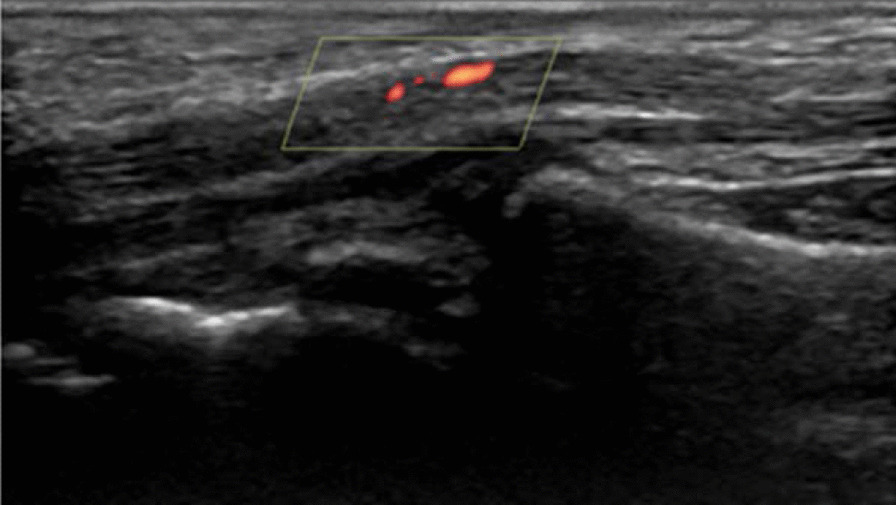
Intraneural blood flow of median nerve depicted by power Doppler at the distal crease level
